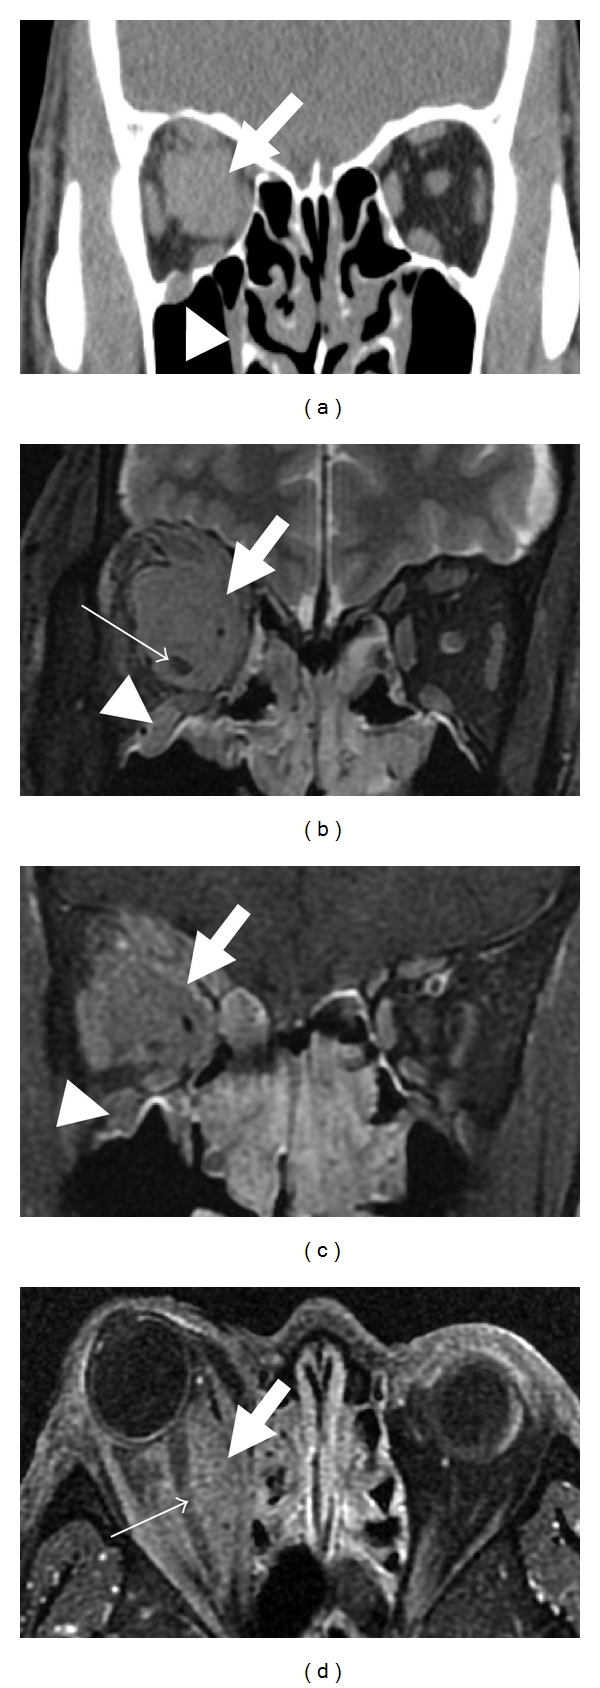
CT (a) and MRI (b: T2-weighted image; (c and d): contrast-enhanced T1-weighted images) of orbital space in 44-year-old man (case 4). Soft tissue mass is detected in the right orbital space (a; arrow). This lesion extends along the right optic nerve in MRI (b–d; arrows). The right optic nerve penetrating the lesion is detectable in MRI (b and d; small arrows). Soft tissue mass along the right infraorbital nerve is also noted (a–c; arrowheads).
